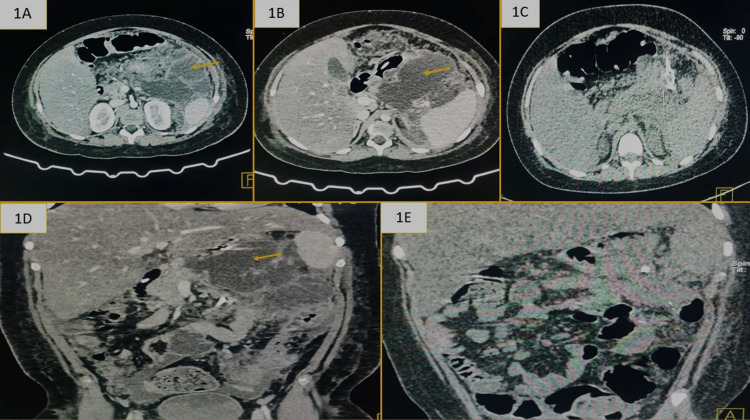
Computed tomography image of one of our patients (A, B) Preprocedural axial CECT images showing well-defined collection involving the distal body and tail of the pancreas extending till anterior pararenal space and large collection in the lesser sac compressing the greater curvature of the stomach. (C) Post-percutaneous catheter drainage axial CT image showing significant reduction in the peripancreatic collection. (D) Pre-procedural coronal CECT showing large collection in the peripancreatic region and left anterior pararenal space. (E) Post-procedural coronal CT shows drainage tube in situ with significant reduction in collection. CT, computed tomography
